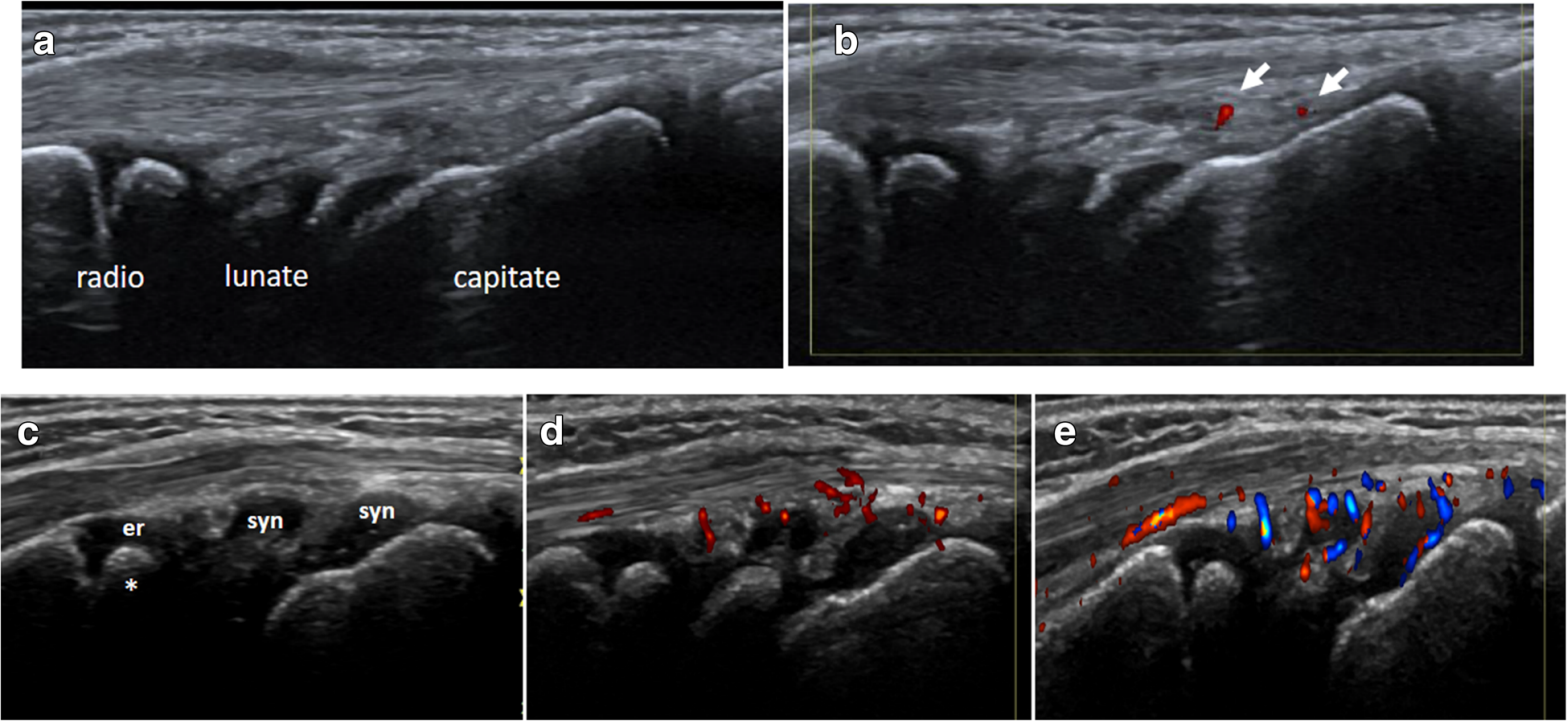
Longitudinal view of the dorsal aspect of the wrist joint in a 6-year-old child. The upper images (a, b) show the normal sonoanatomy on Grey-scale US (a) and power-Doppler US (b) showing single vessels close to os capitate. The lower images (c, d, e) show synovitis of the wrist joint in a patient with JIA. Synovial vascularity detected in the joint recesses by Doppler (image d, power Doppler and image e, color Doppler) reflects active inflammation. The distal epiphyseal cartilage of radius (er) is visible as an anechoic structure surrounding the secondary ossification nucleus (*). Dynamic examination let distinguish the epiphyseal cartilage of radius from effusion/synovitis (syn)
